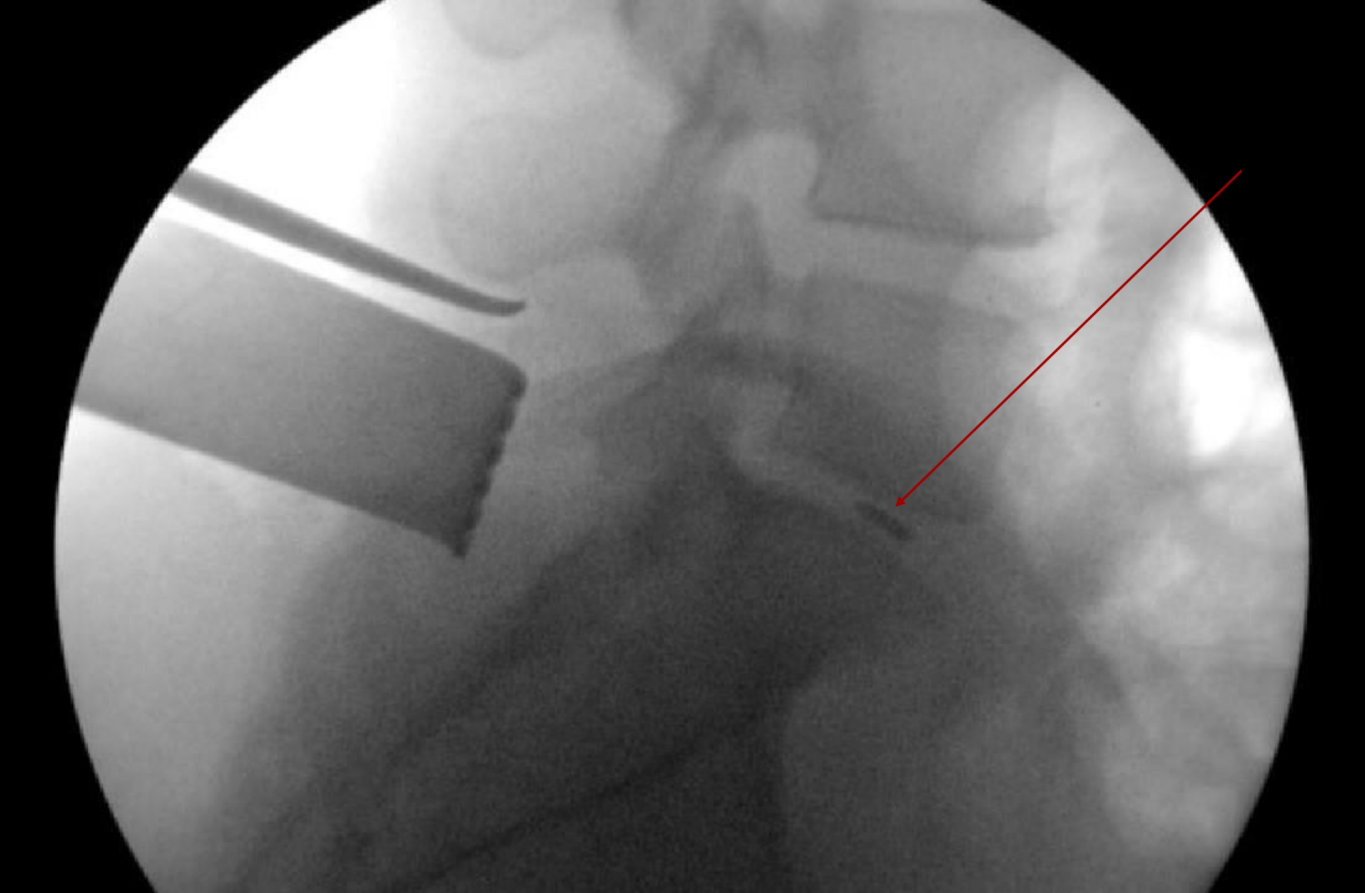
Lateral Radiograph of Broken Pituitary Disc Rongeur
